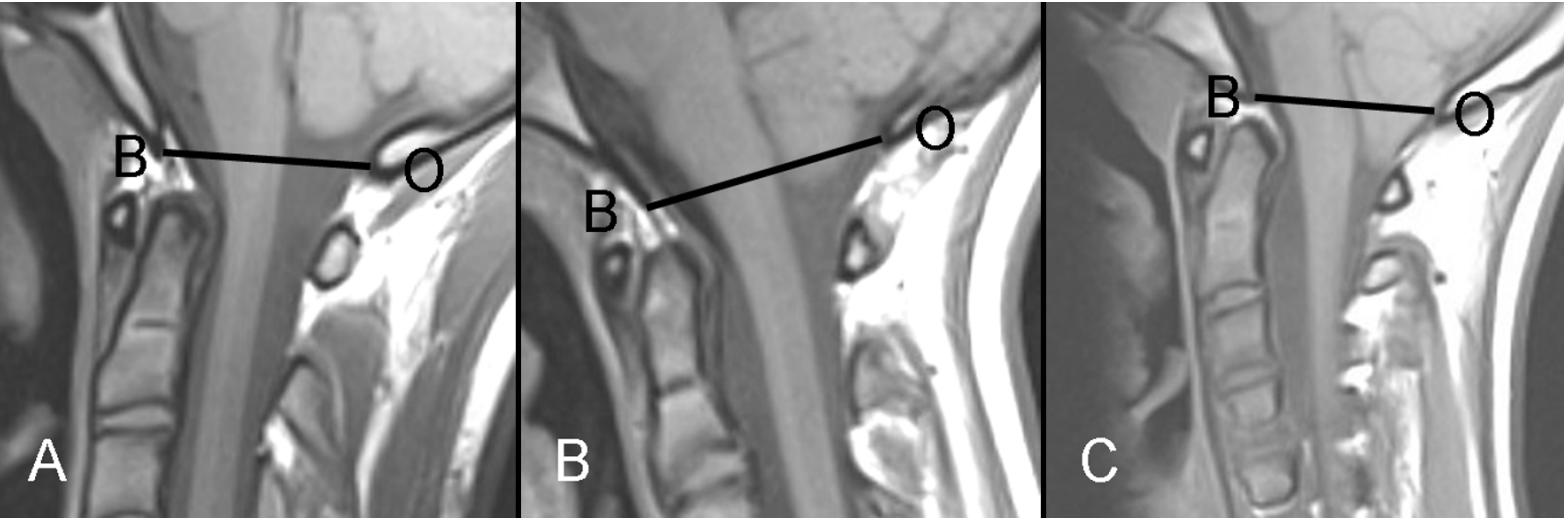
(A-C): MRI sagittal midline T1-weighted images of three different patients with scoliosis. The reference lines (BO-lines) connect the basion (B) and opisthion (O). (A) 16 years old boy with IS and no cerebellar ectopia. The cerebellar tonsils are above the BO-line. (B) 16 years old girl with IS showing descent of cerebellar tonsils 4 mm below the BO-line (tonsillar ectopia). (C) 14 years old girl with scoliosis, and Chiari I malformation showing cerebellar tonsils 8 mm below the BO-line (Chiari malformation).
